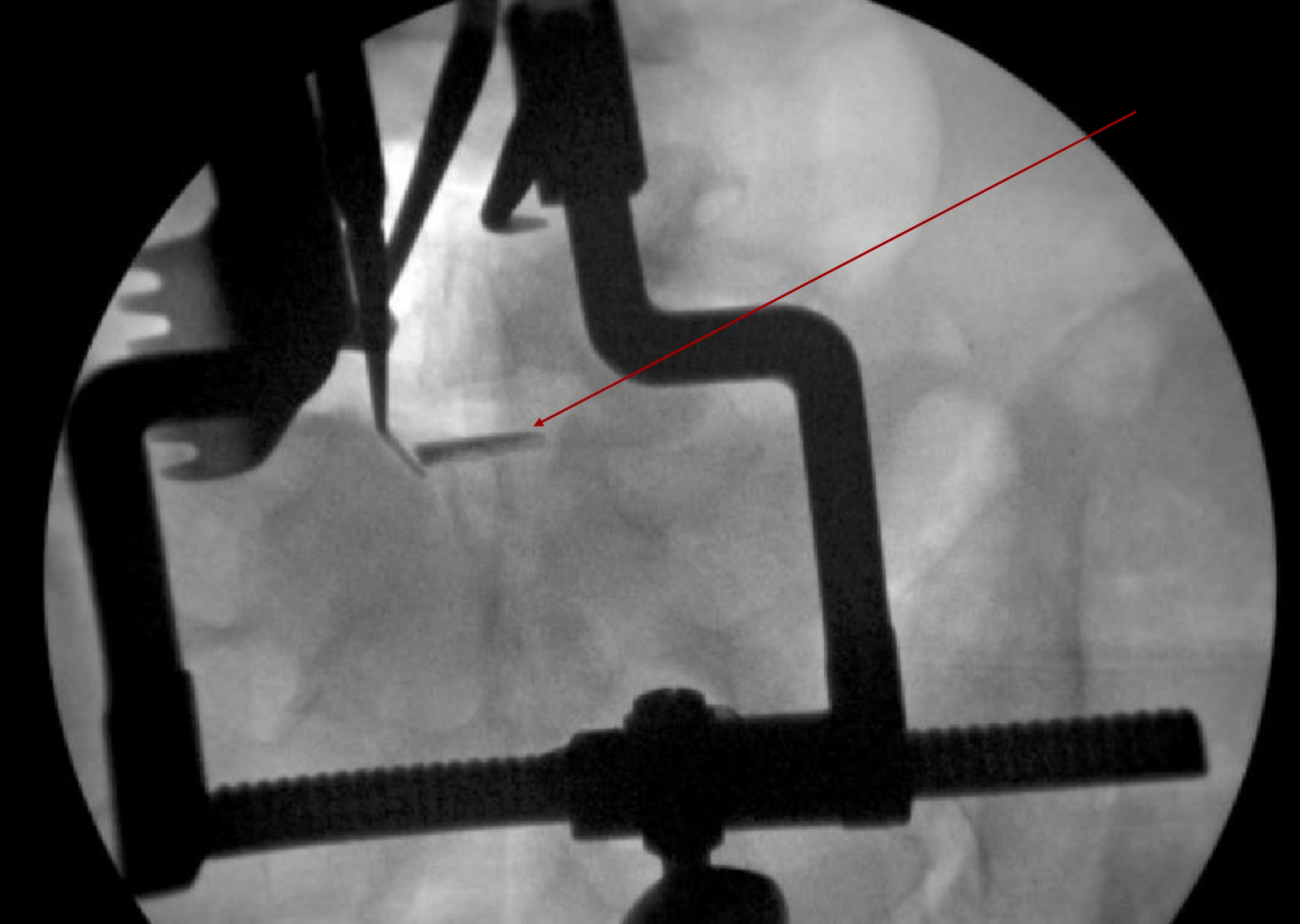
Anterior Posterior Radiograph of Attempted Removal of Broken Rongeur
